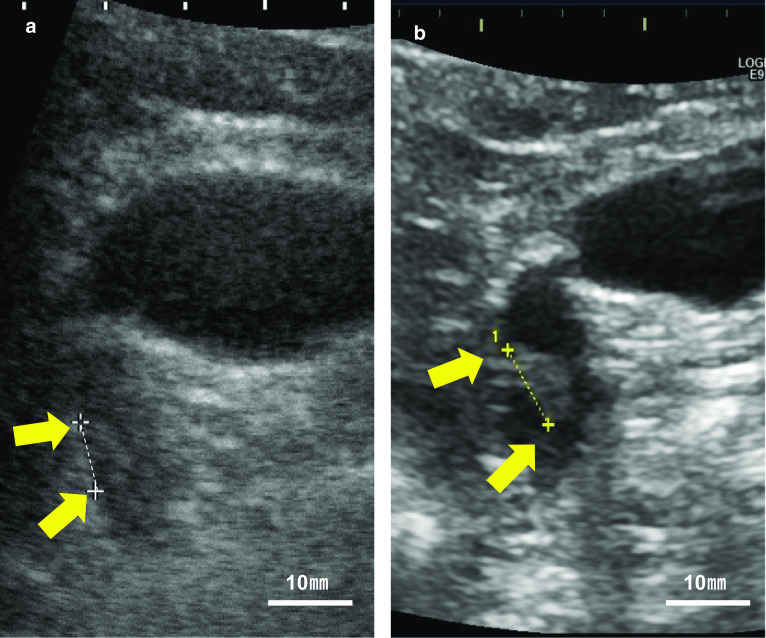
Ultrasound of the gallbladder showed a well-defined, smooth mass of mixed hypo- and iso-echogenicity at the gallbladder neck (arrows). a The diameter of the mass was 8.3 mm. b The tumor increased in size to 9.9 mm. Scale bar = 10 mm
